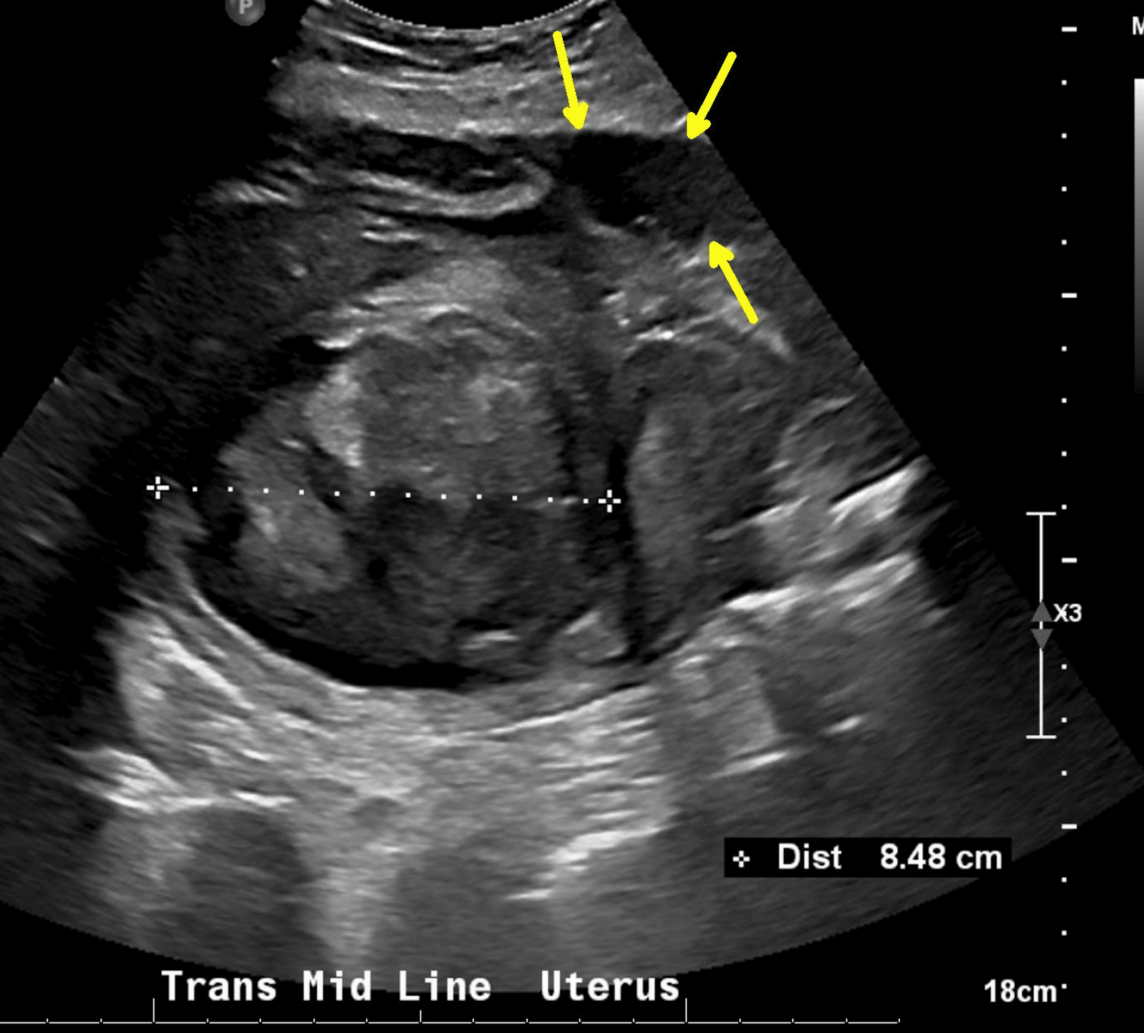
Transverse image of pelvic ultrasound (day 54 after initial admission and 39 days after cesarean delivery). The area between the yellow arrows represents fluid above the uterus in the region of the cesarean incision, measuring approximately 4 cm x 2.5 cm. The uterine wall with a myoma measures 8.48 cm and is between the cursors.
